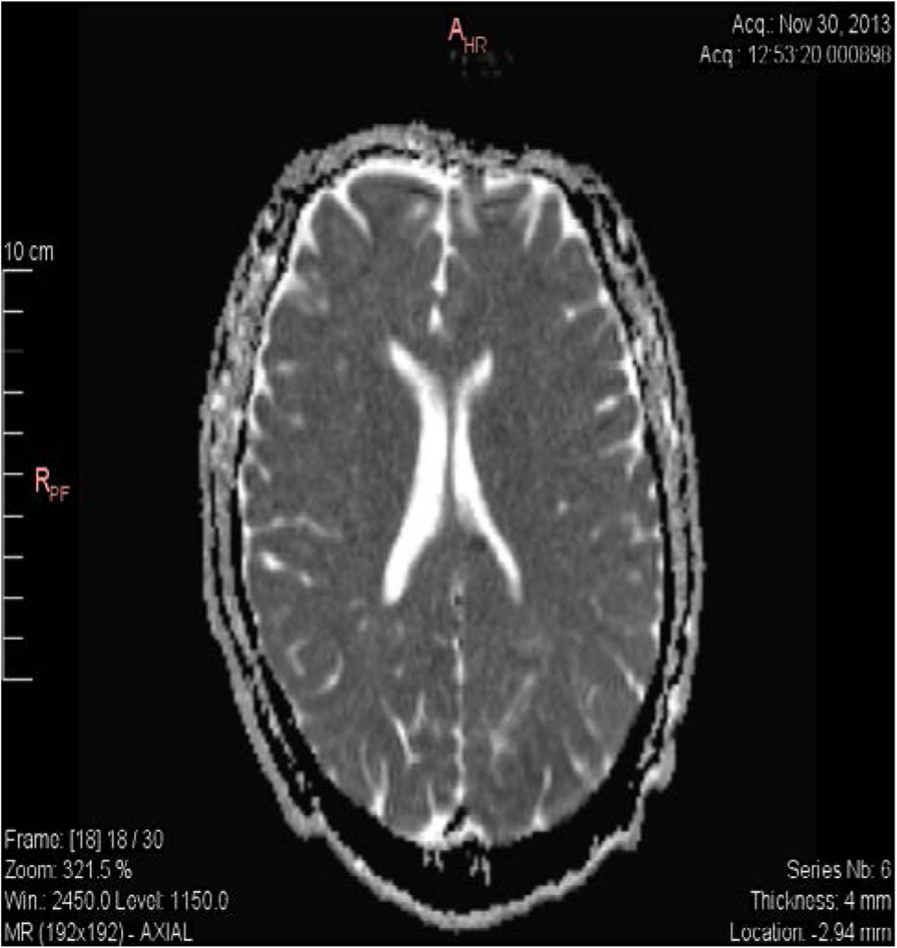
MRI showing small punctiform lesions in subcortical areas of ischemic origin
